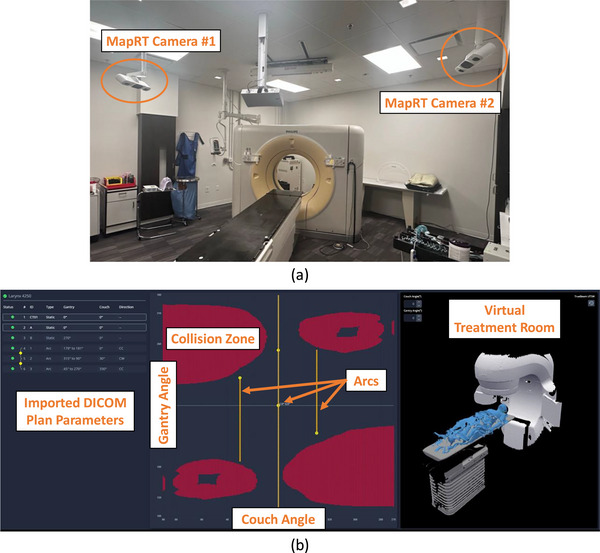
(a) The MapRT camera setup in the CT vault at our institution and (b) the interactive MapRT clearance mapping system interface.
